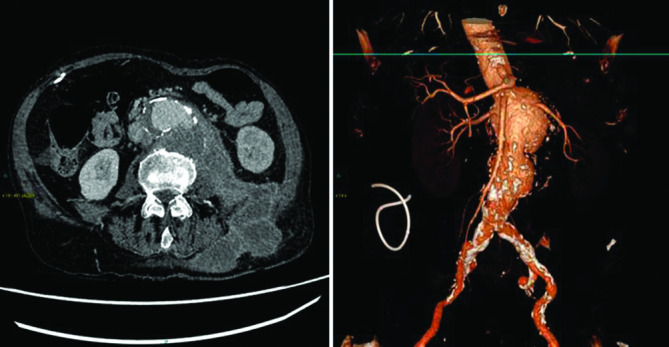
Axial CT and volume rendering technique (VRT) showing ruptured peri-visceral AAA with associated retroperitoneal collection tracking into the subcutaneous layer and showing rim enhancement.
